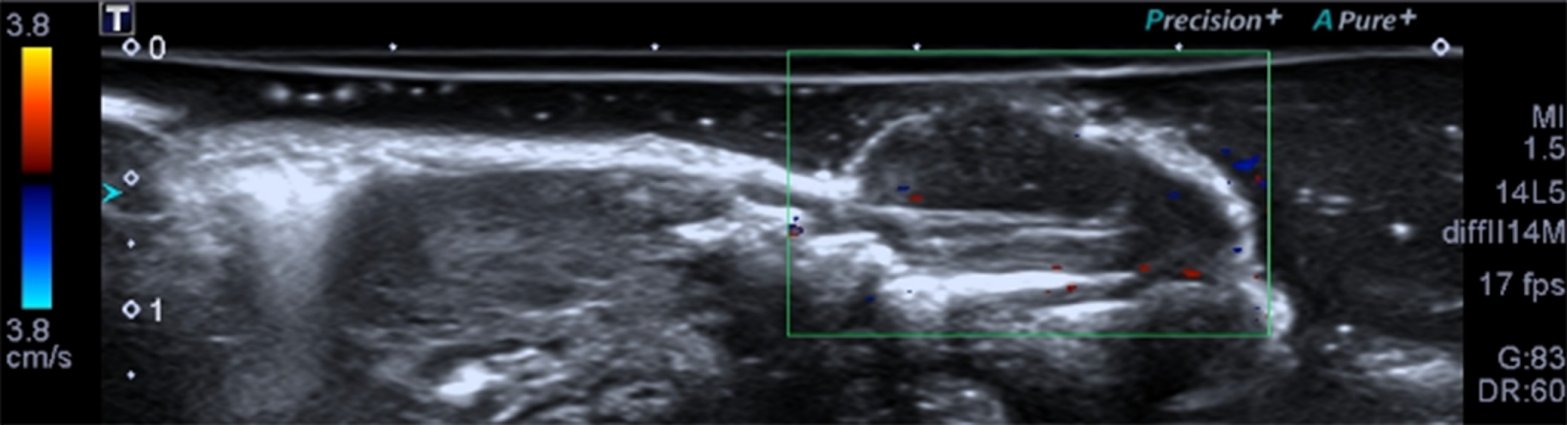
Color Doppler detection of NPC.
